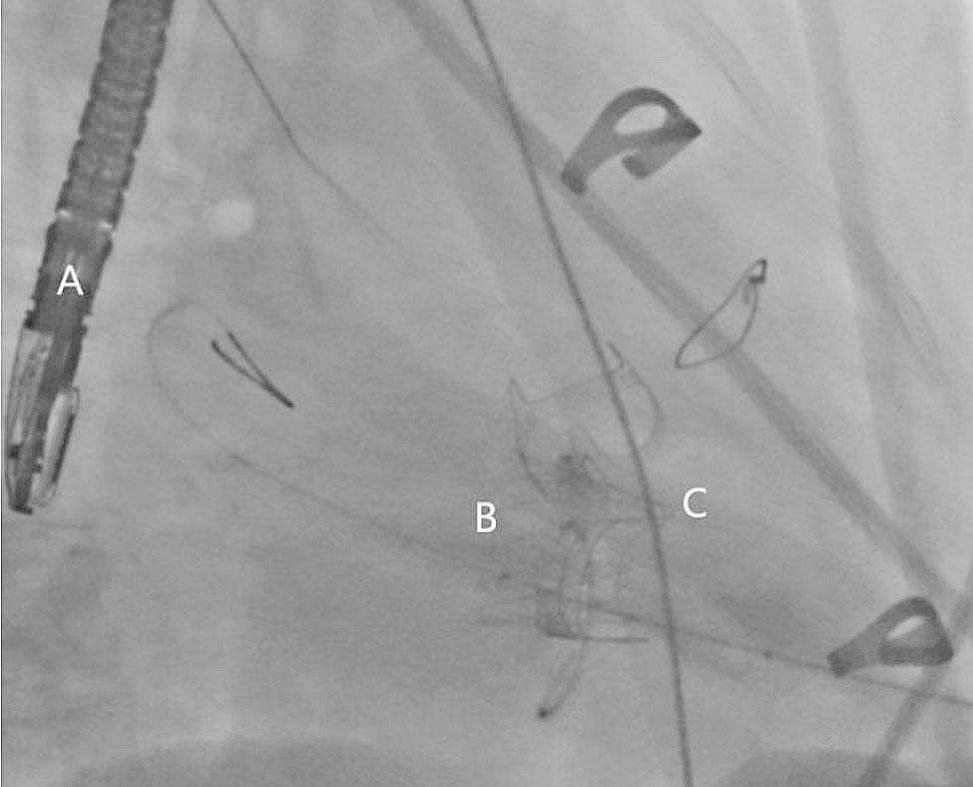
Fluoroscopic image depicting (A) transesophageal echocardiogram probe, (B) 25 mm J-valve, and (C) mitral bioprosthetic valve failure
